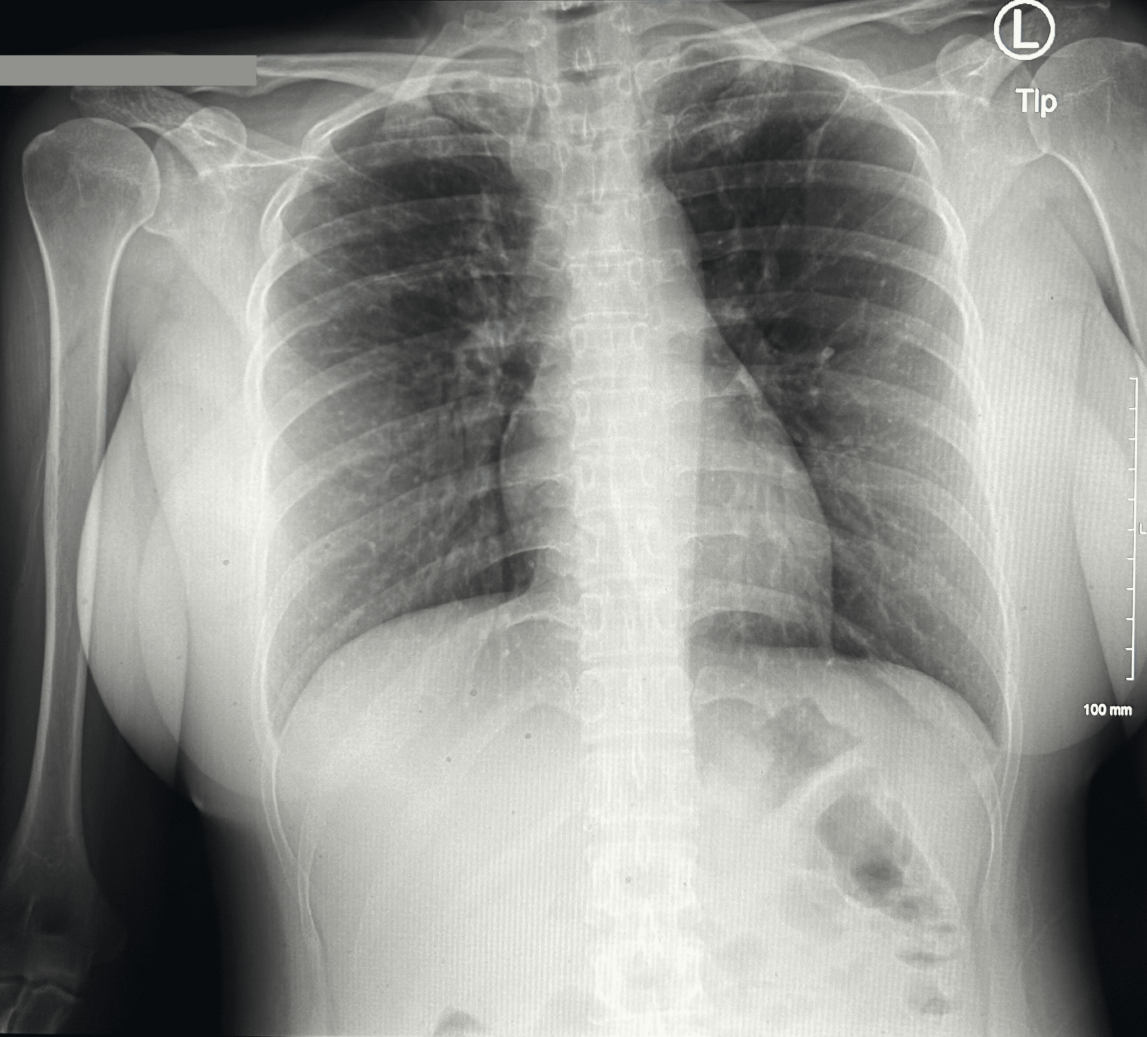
Chest X-ray demonstrating no evidence of active TB TB, tuberculosis
